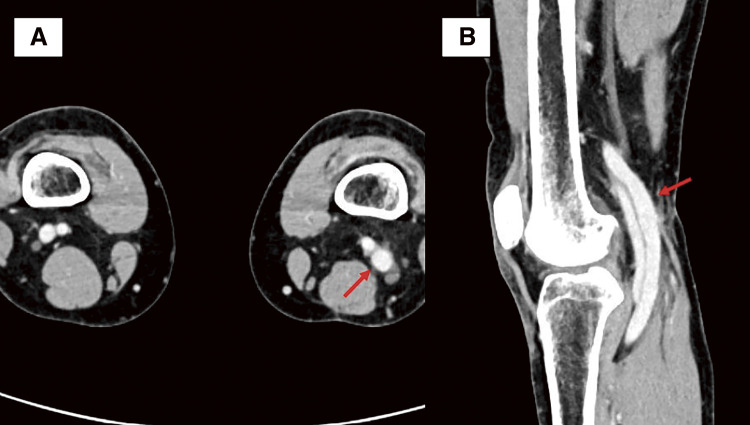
Fig. 3 Postoperative contrast-enhanced CT. (A and B) CT reveals no thrombus, coarctation, or other abnormalities. CT: computed tomography
